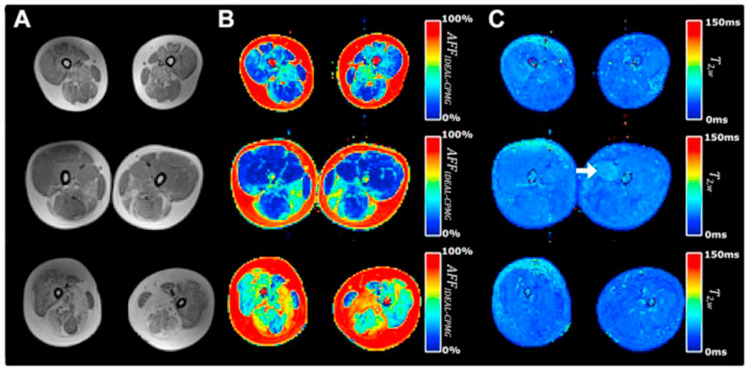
Representative T1-weighted and IDEAL-CPMG images of the thigh muscles in three subjects with DMD. A T1-weighted image (A), T2-corrected fat fraction map (B), and water T2 map (C) are shown representing subject anatomy, changes in muscle apparent fat fraction (AFFIDEAL-CPMG), and muscle water T2 (T2w IDEAL-CPMG), respectively, in the thigh muscles of subjects with DMD. Different severity of fatty degeneration is present in the thigh muscles of each subject, whereas inflammatory activity is sparse and seen in only few muscles (arrow). Reproduced with permission from the authors in Ref. [107].
